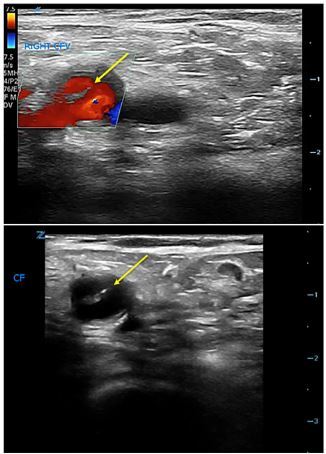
Grayscale and color ultrasound demonstrating intimal flap (arrow) in the right common femoral artery.
